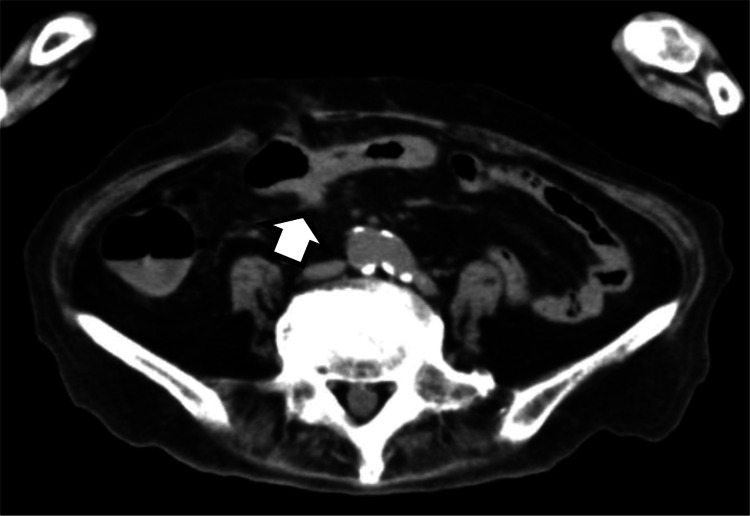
Computed tomography findings on day 106. By day 106, edema of the transverse colon and other abnormalities, including extraluminal feces, which were demonstrated in Figure 1B had resolved, and a small residual soft-tissue density persisted (arrow). See Figure 1B (arrow “s”). This CT was taken at the almost identical slice position as Figure 1B.
